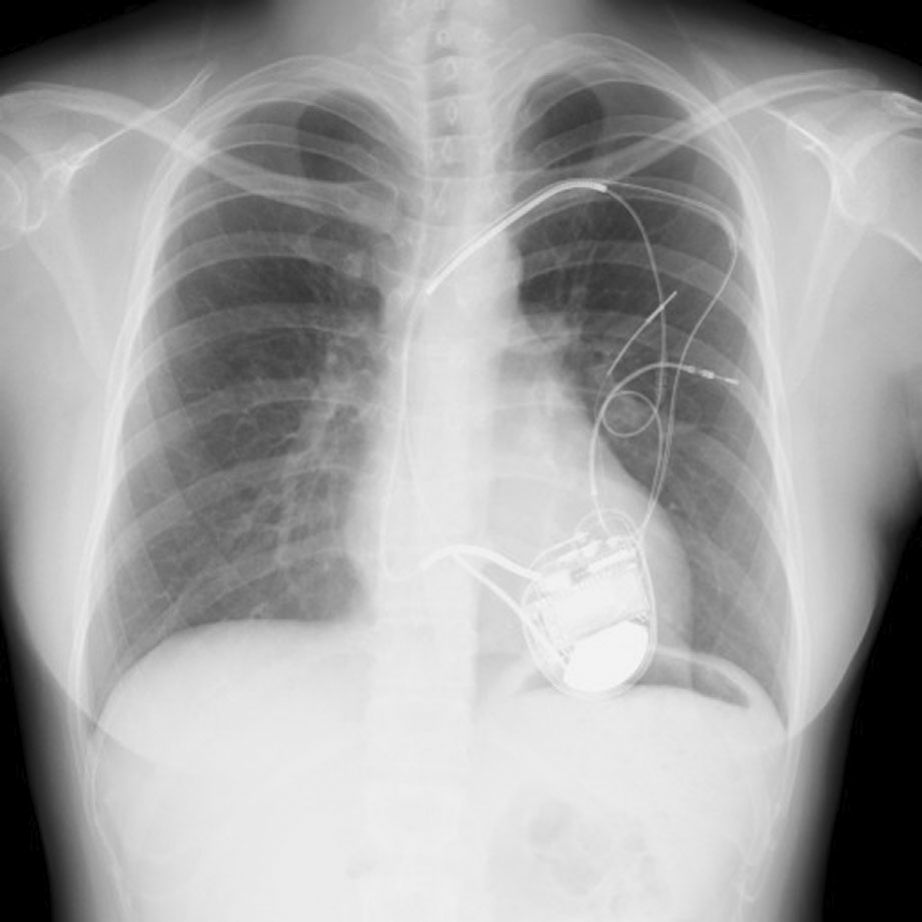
Chest radiograph. No pulmonary abnormalities were present
